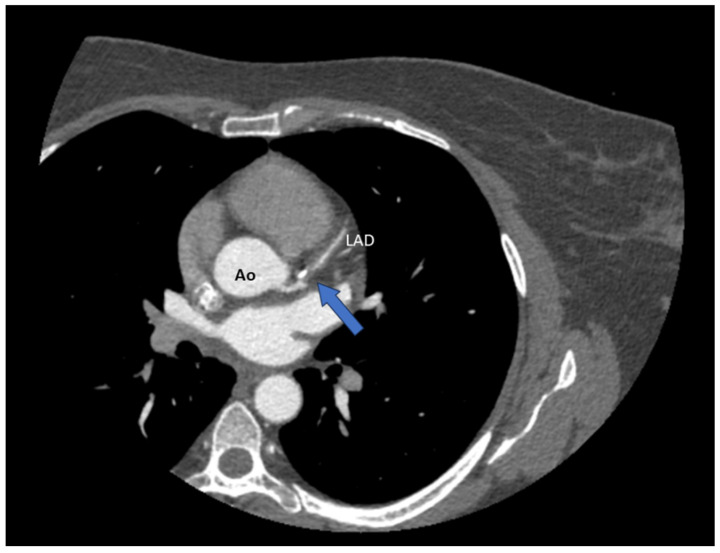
Seeing as the patient had a low suspicion for atherosclerotic disease, we first performed a CT coronary angiography (CTCA), which revealed a significant stenosis of the left anterior descending artery (LAD) (blue arrow); Ao = aorta.
